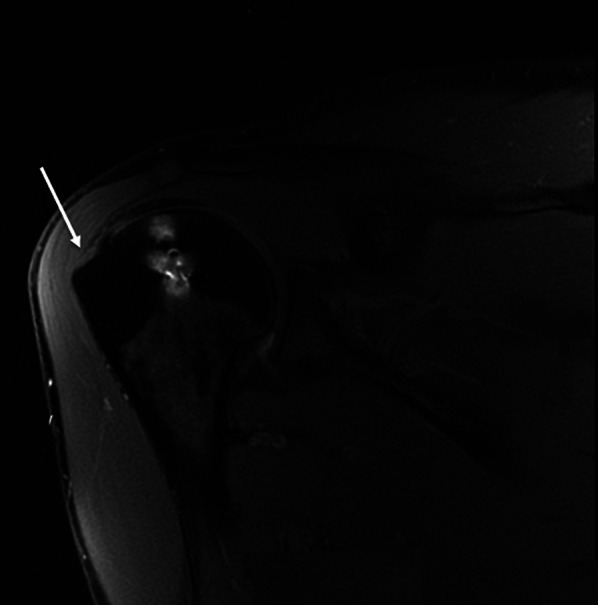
MRI coronal cut of the shoulder of a patient who underwent the remplissage procedure: the* white arrow* shows a partial tear, articular and bursal, of the supraspinatus tendon
